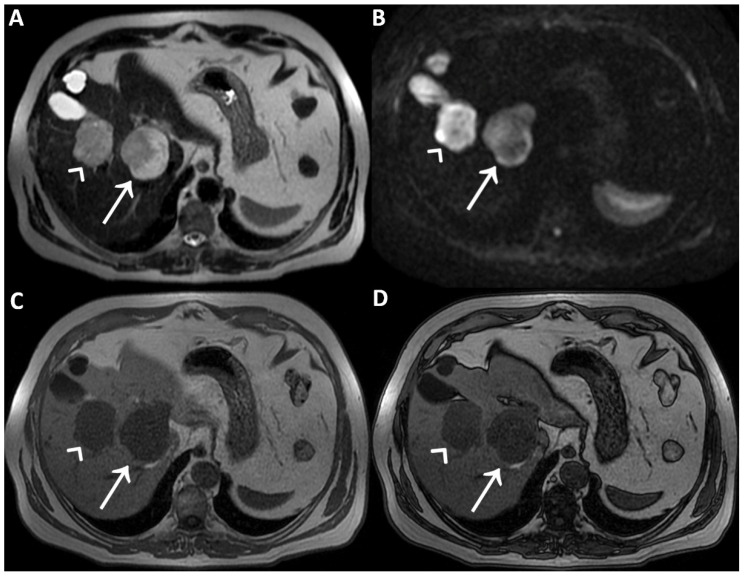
A man in his early 60s with unremarkable medical history and normal laboratory data underwent an abdominal ultrasound for insurance reasons, which detected two liver lesions, the largest one localized deeply in the hepatic parenchyma. Due to the patient’s high body mass index, which reduced the ultrasound’s diagnostic quality, panoramic imaging was required to achieve the final diagnosis. Therefore, the patient underwent liver Magnetic Resonance Imaging (MRI) performed with hepatospecific contrast agent (Gd-EOB-DTPA). MRI was performed by using a 1.5 T superconducting system (Signa; GE Medical Systems, Milwaukee, Brookfield, WI, USA) with a body phased-array multicoil for signal detection. The two liver lesions appeared to be inhomogeneously hyperintense on T2-Weighted Images (T2WI) (straight arrow and arrowhead in (A)) and on Diffusion-Weighted Images with b-value = 800 (DWI) (straight arrow and arrowhead in (B)) and homogeneously hypointense on T1-Weighted Images (T1WI) (straight arrow and arrowhead in (C), in-phase T1WI, and (D) opposed-phase T1WI). It was not possible to establish the benignity of the two liver lesions evaluating T2WI and DWI acquired after contrast agent administration, in particular due to the inhomogeneous signal intensity shown on T2WI. To ascertain that it had not been caused by the contrast-induced reduction in the hydrogen nuclei relaxation time, it was necessary to re-acquire the same images without previous contrast administration a few days later.
